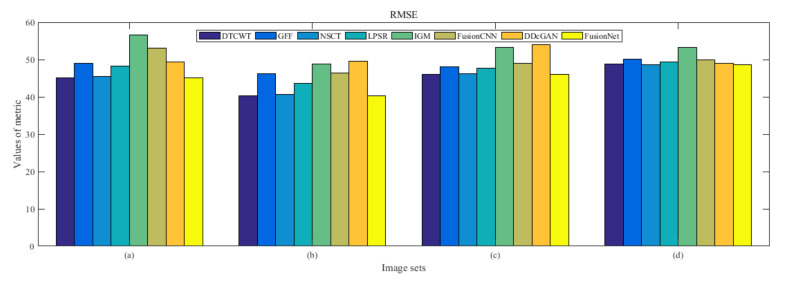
Values of root mean squared error (RMSE) in the fused images (MRI-FDG): (a) is composed of eight images in Figure 25, (b) is composed of eight images in Figure 26, (c) is composed of eight images in Figure 27, (d) is composed of eight images in Figure 28.
